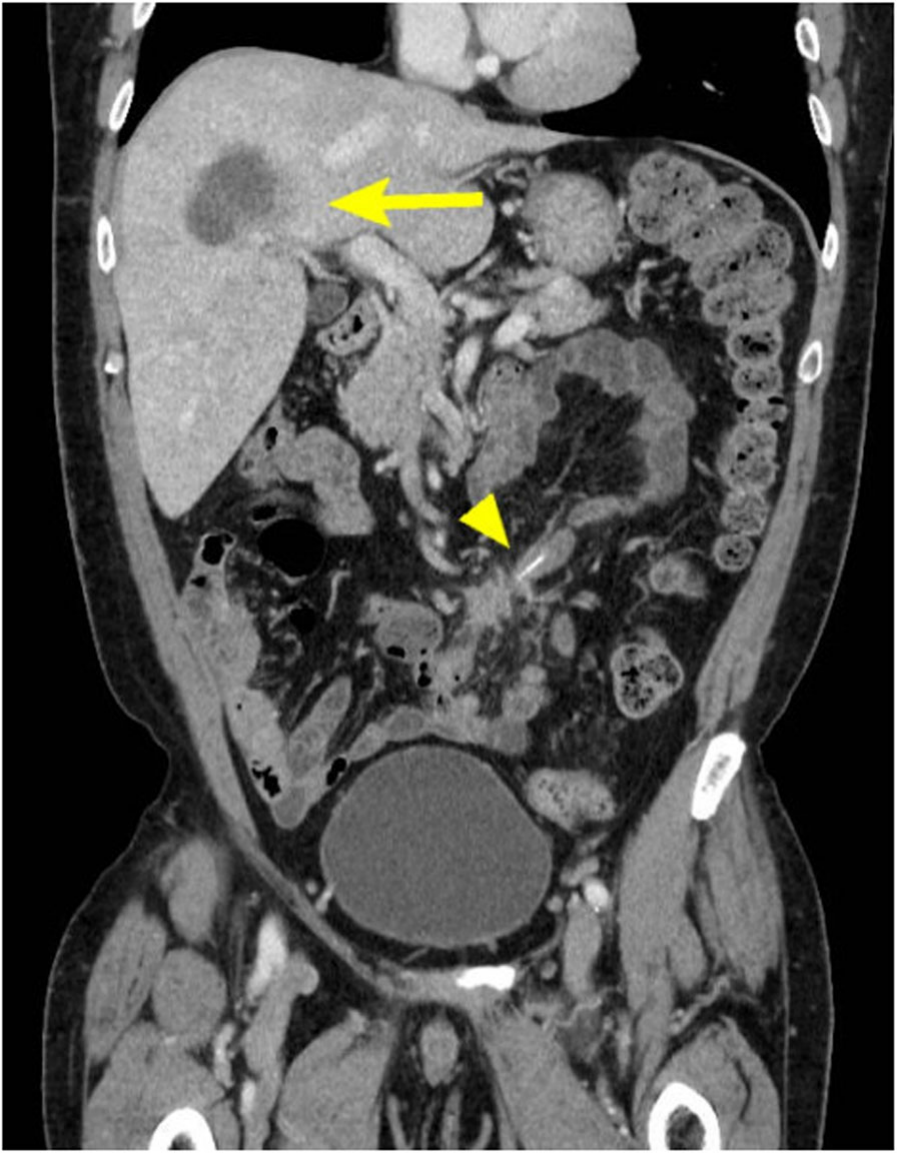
Enhanced computed tomography (CT) revealed an abscess in the right lobe of the liver and a needle-like foreign body in the small intestine with fat stranding
